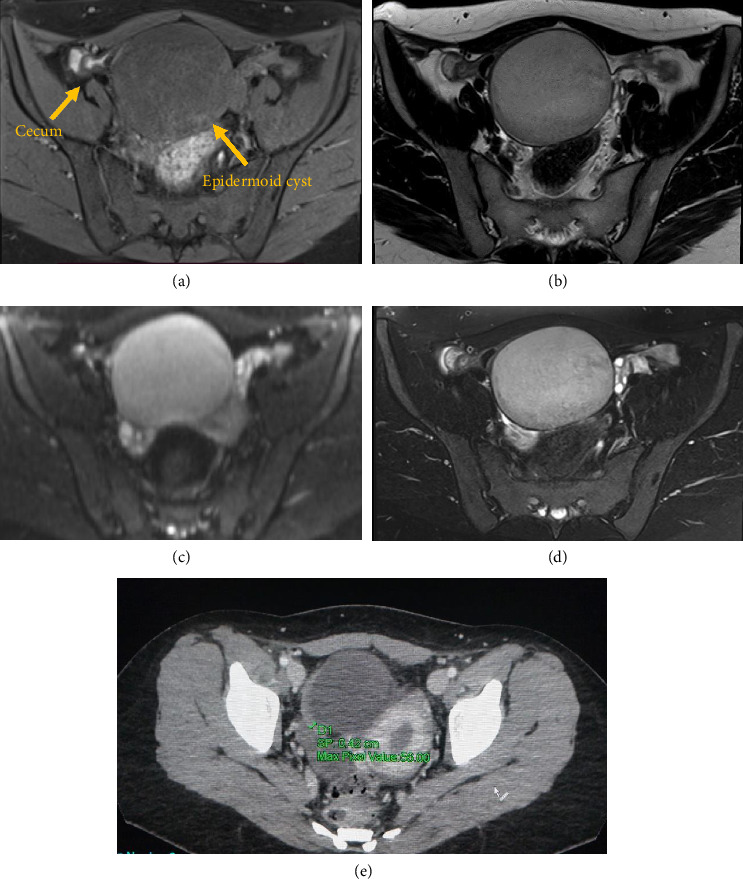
Abdominal MRI. MRI reveals a cystic mass attached to the cecum, appearing hypointense on T1-weighted imaging (a) and hyperintense on T2-weighted imaging (b–d). (a) Axial MRI image T1 TSE Dixon water only of the epidermoid cyst. (b) Axial MRI image T2 TSE, with the fluid and internal debrides of epidermoid cyst. (c) Axial MRI image DWI, b 800, shows the high restriction. (d) Axial MRI image, contrast-enhanced T2 TSE, demonstrates the lack of enhancement. (e) CECT abdomen reveals the appendix of normal dimension (4 mm).
